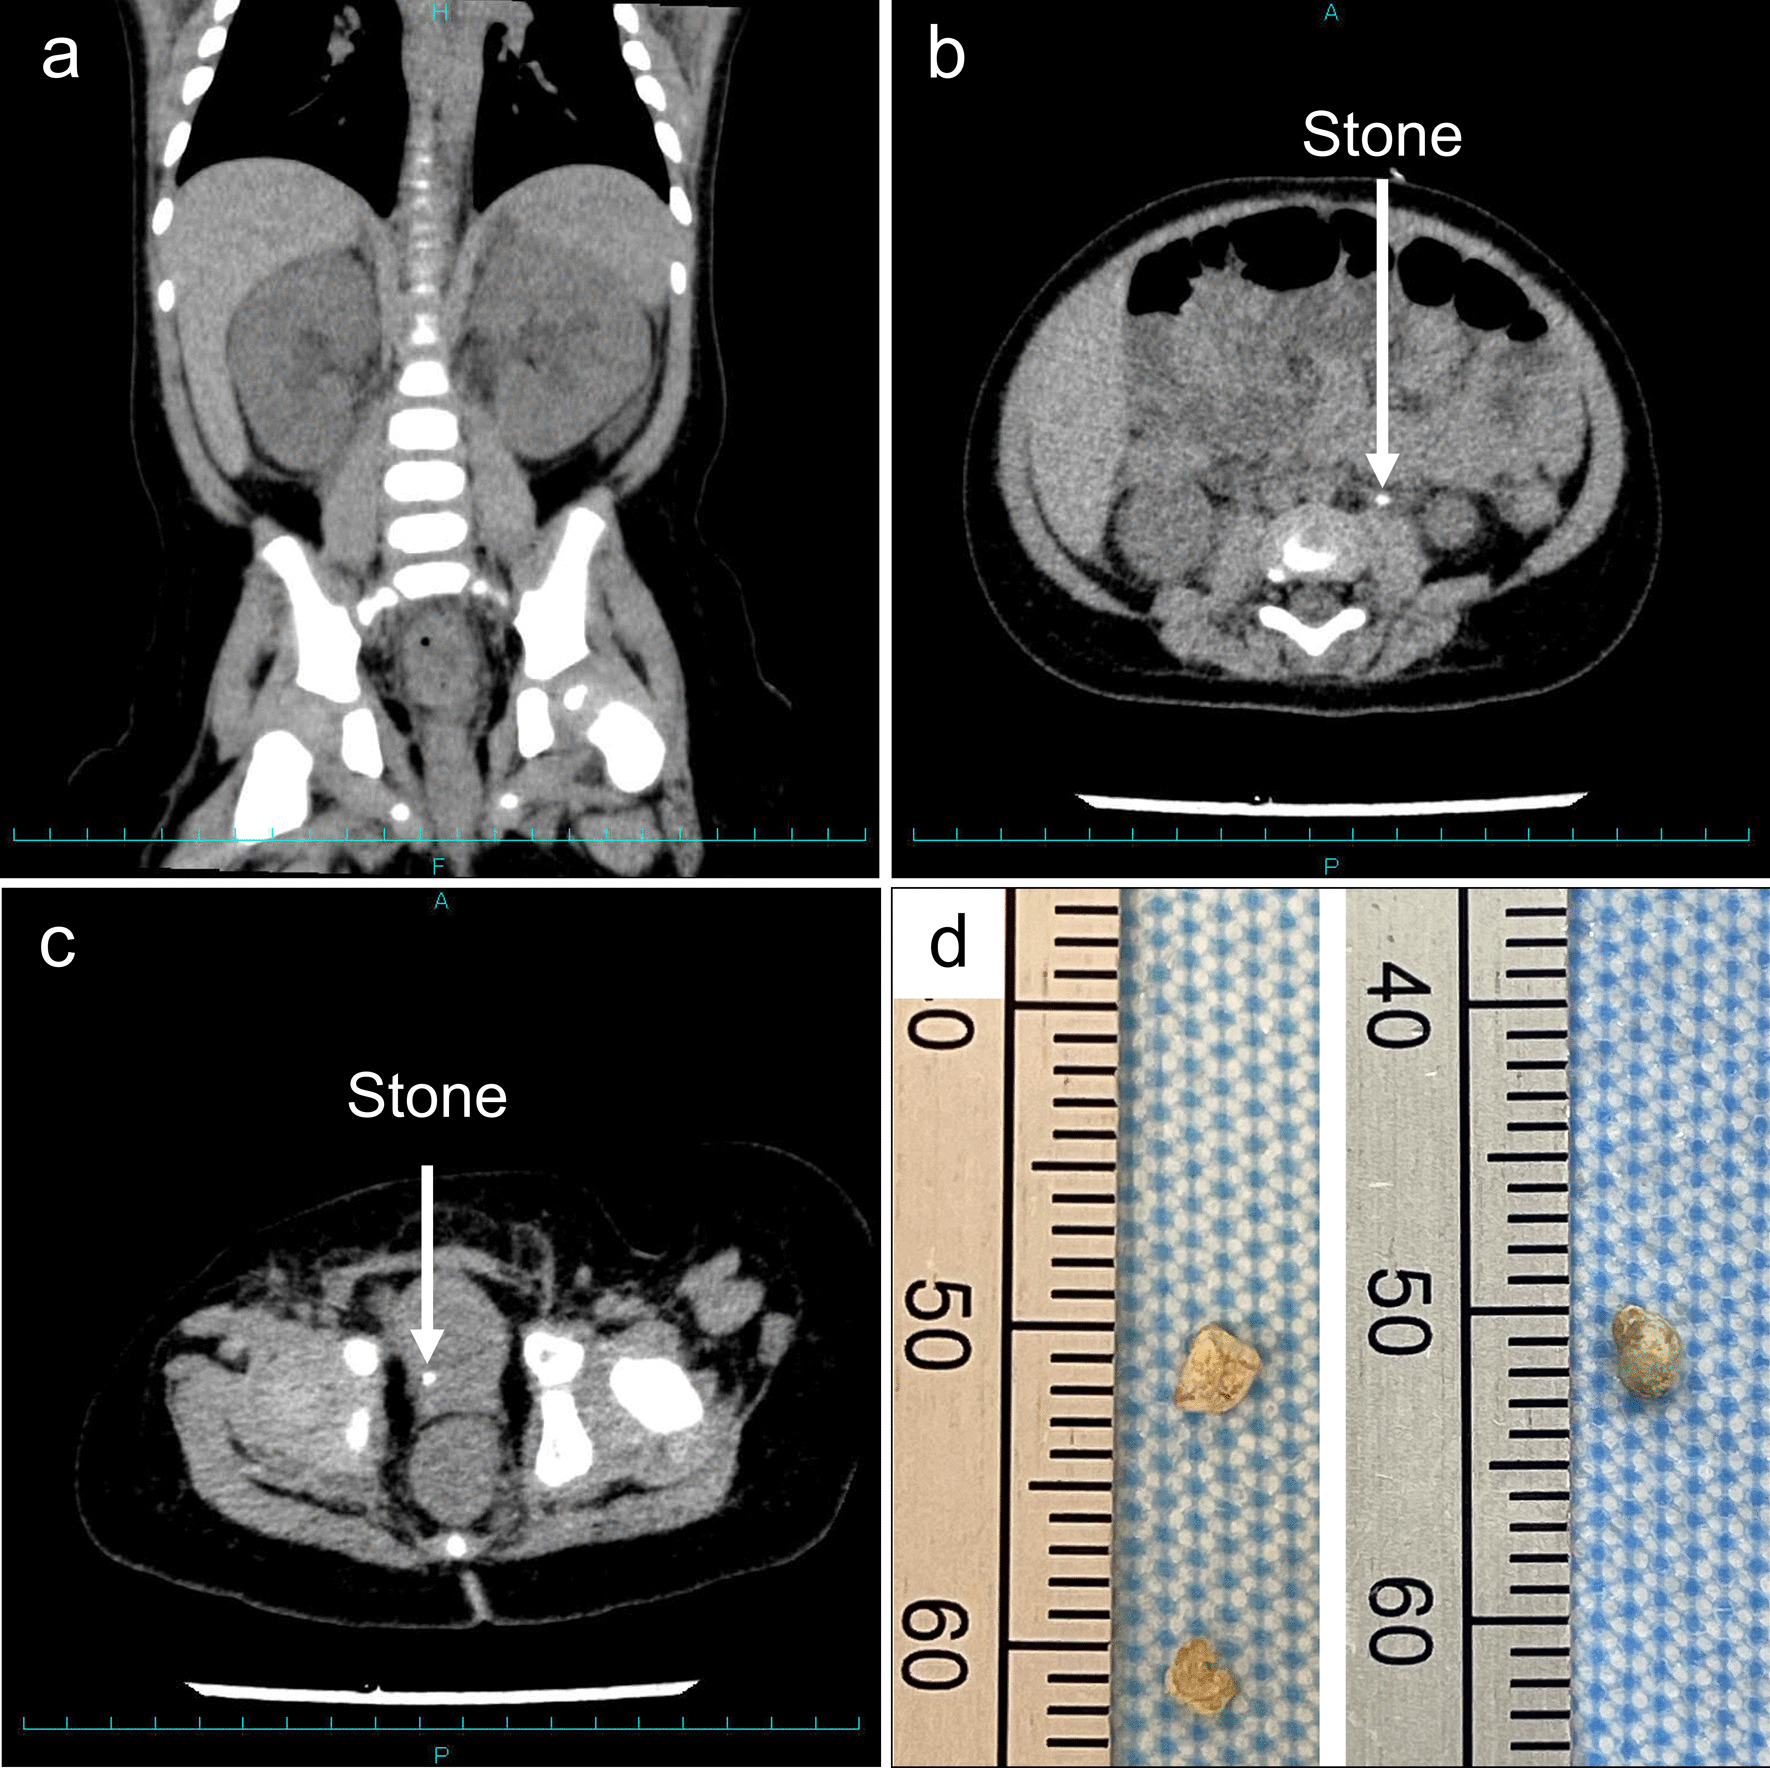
a Coronal computed tomography (CT) section reveals hydronephrosis. b Transverse CT section shows left ureteral stones (arrows). c Transverse CT section reveals right bladder ureteral junction stones (arrows). d A few stones with a size of 2–3 mm that were spontaneously excreted
